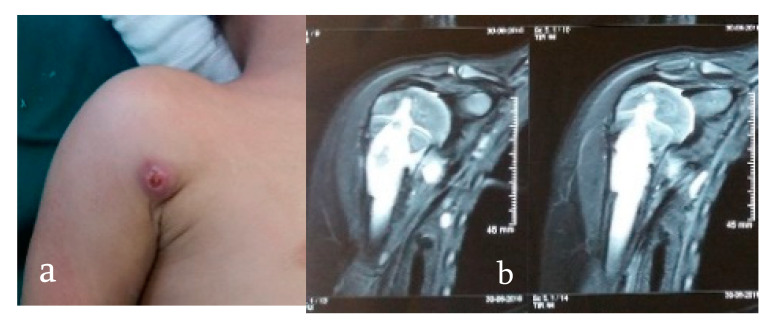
(a) An 11-month-old boy presents with discharging sinus over right anterior axillary fold with restricted and painful movement of his right shoulder. (b) Magnetic resonance imaging (MRI) reveals infective lesion crossing the physeal plate of the proximal humerus.
